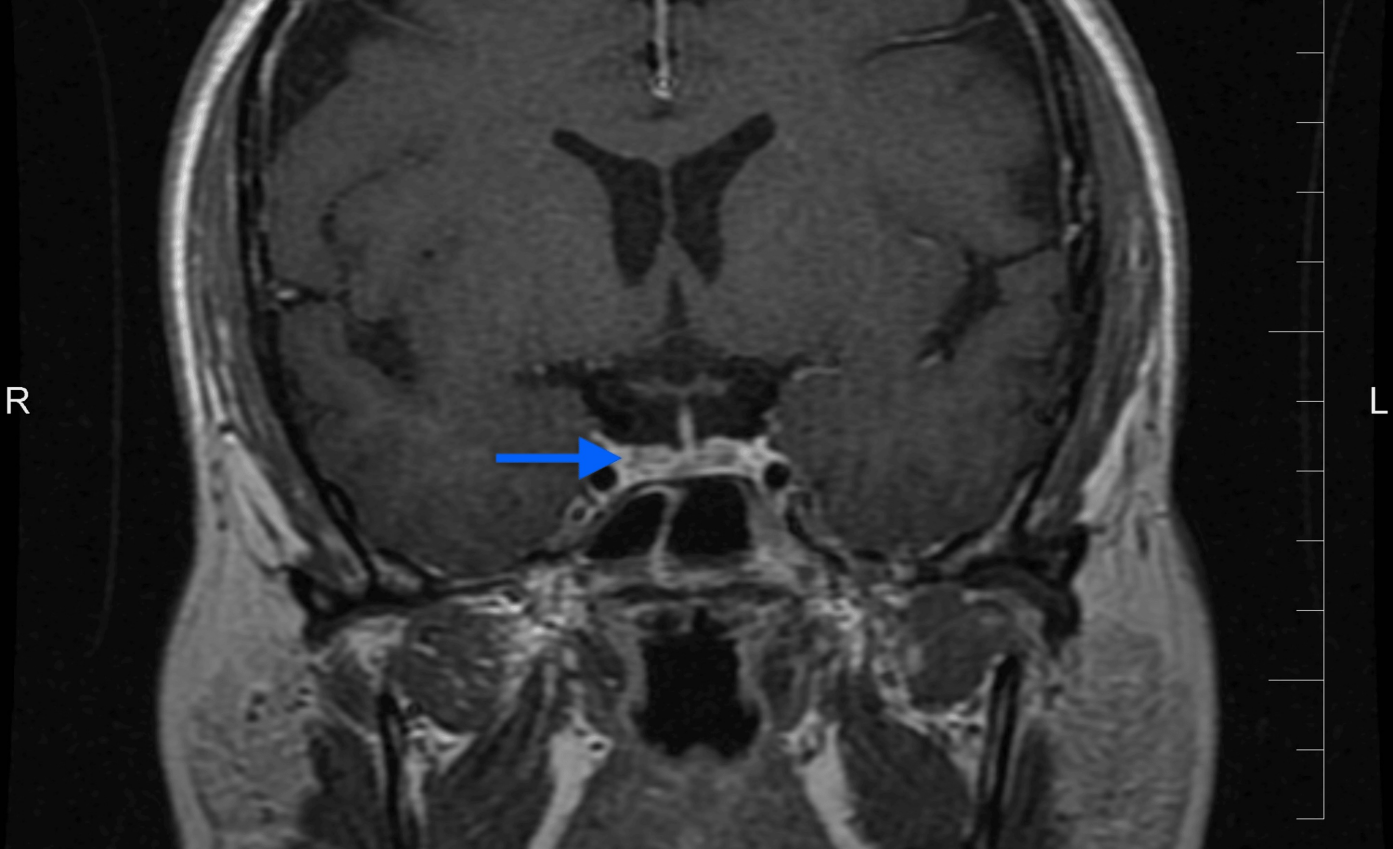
Coronal T1-weighted post-contrast MRI demonstrating postoperative changes at the prior adenoma resection site (blue arrow)
